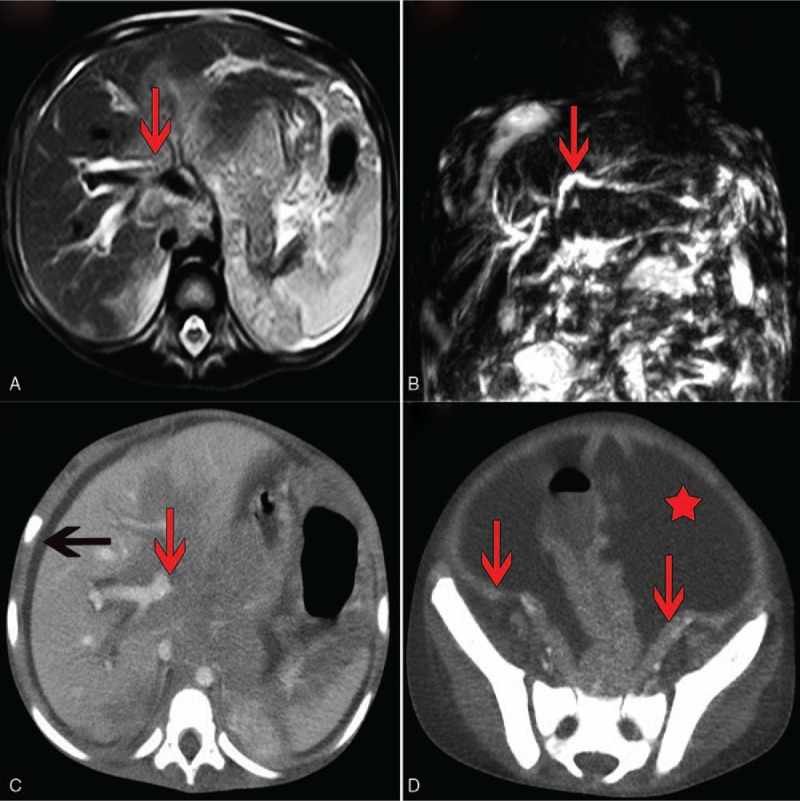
Imaging results magnetic resonance cholangiopancreatography (MRCP) showed lymph node enlargement and fusion in the hilar region, with a slightly high-density shadow (A) as well as mild dilatation of the intrahepatic bile duct (red arrow), no expansion of the extrahepatic bile ducts, and no manifestation of the gallbladder or common bile duct (B). Abdominal contrast-enhanced computed tomography (CT) showed enlargement of lymph nodes in the hilar region (red arrow) and perihepatic effusion (black arrow) (C) as well as a large amount of ascites (red asterisk) and diffuse incrassation in the peritoneum (red arrow) (D).
